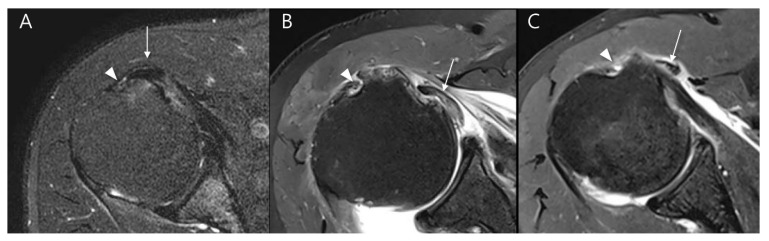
Evaluation of long head of the biceps tendon (LHBT) instability on T2-weighted oblique axial fat-suppressed images in relation to the bicipital groove: (A) LHBT (arrow) is partially displaced to the medial margin of the bicipital groove (arrowhead) without complete dislocation, consistent with subluxation. (B) LHBT (arrow) is completely displaced medially from the bicipital groove (arrowhead) and lies within the glenohumeral joint space, beneath the subscapularis tendon, representing an intra-articular dislocation. (C) LHBT (arrow) is completely displaced medially from the bicipital groove (arrowhead) and is located outside the joint capsule, overlying the subscapularis muscle, representing an extraarticular dislocation.
